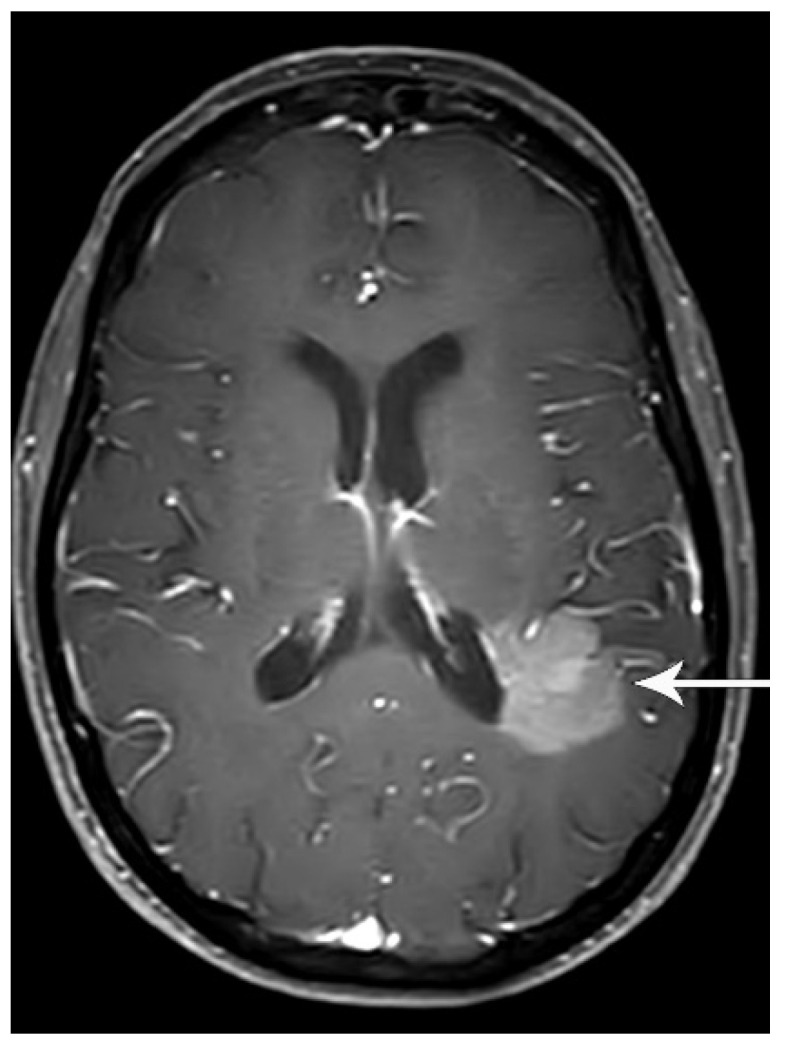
M1b disease, single extrathoracic metastasis. Contrast-enhanced brain MRI shows a left parietal enhancing metastasis (arrow). A single focus of extrathoracic metastasis is M1b disease, which constitutes stage IVA.
